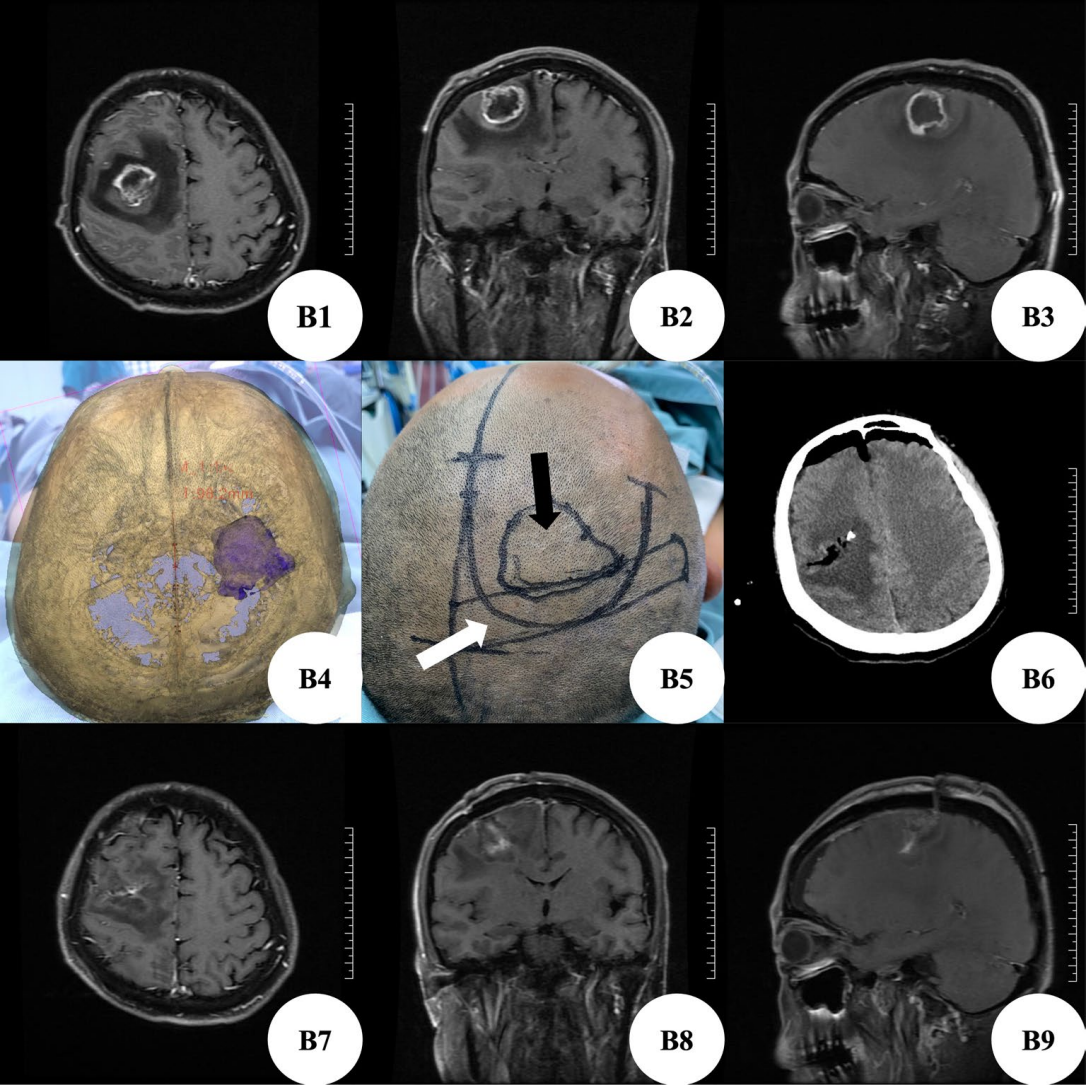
(B1–B3) Enhanced brain MRI showed space occupying lesions in the right frontal lobe; (B4) use the Apple mobile phone APP MosoCam for projection positioning; (B5) the surgical incision was designed according to the projection location. The black arrow was the body surface projection of the tumor, and the white arrow was the body surface projection of the central anterior gyrus; (B6) postoperative brain CT showed that the tumor was resected cleanly without delayed bleeding; (B7–B9) on the 7th day after operation, brain MRI enhanced examination showed that there was no residual tumor.
